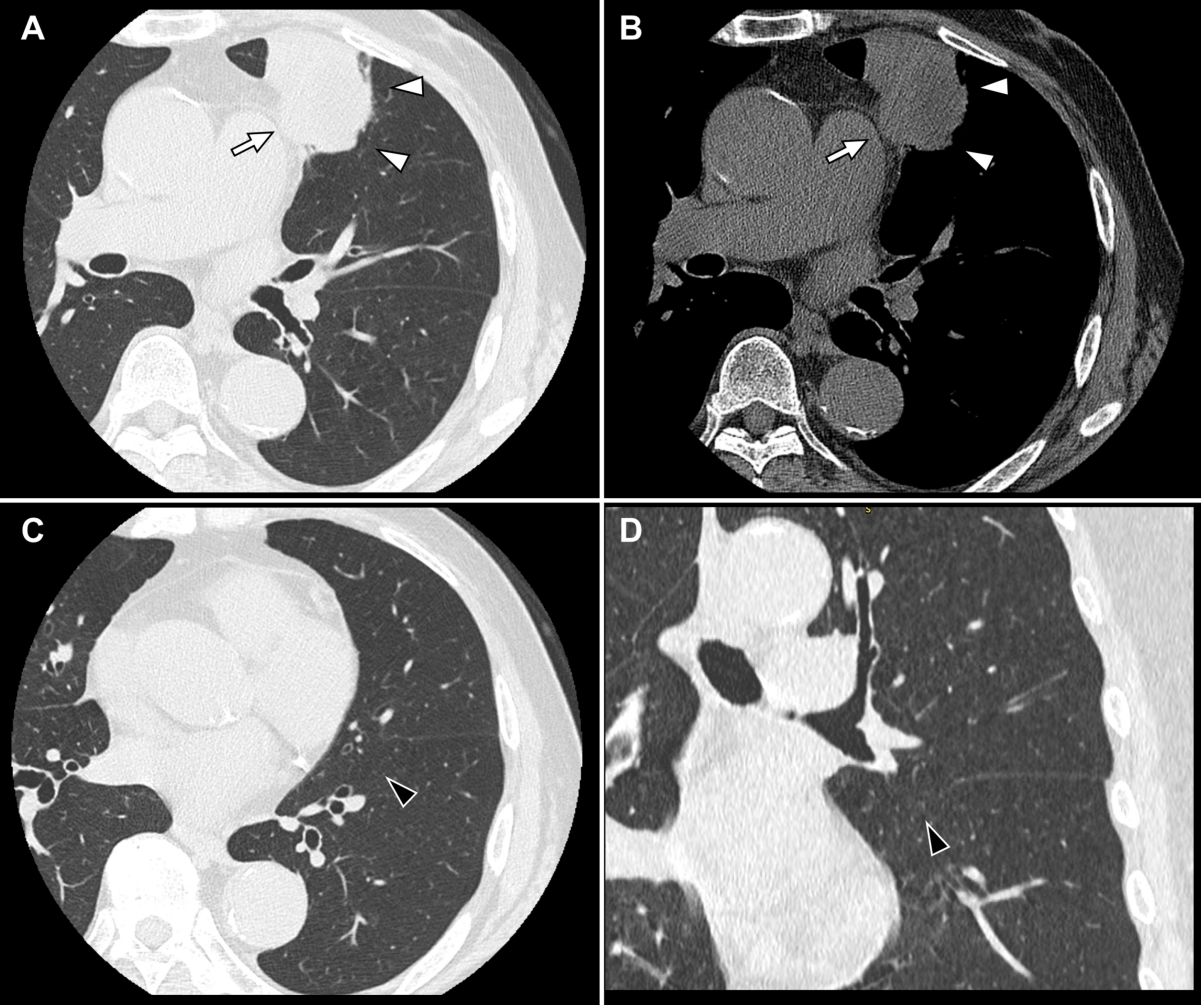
Preoperative chest computed tomography findings. Axial scans (A: lung window; B: mediastinal window) show a 52-mm mass (white arrowheads) in the left upper lobe with suspected invasion of the mediastinal fat (white arrows). An incomplete fissure (black arrowheads) is identified between the lingular and basal segments on axial (C) and coronal (D) images.
